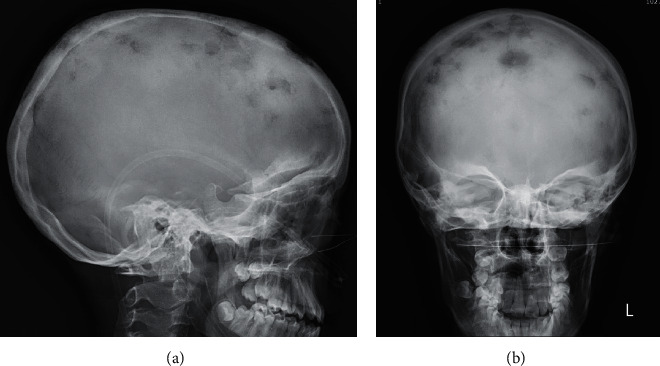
(a) and (b) Skull X-ray of the 13 years old girl with MSMD from Ethiopia: multiple trans-diploic lesions with inner and outer calvarial bone destruction; findings were suggestive of delayed/arrested sphenoid sinus pneumatization.
